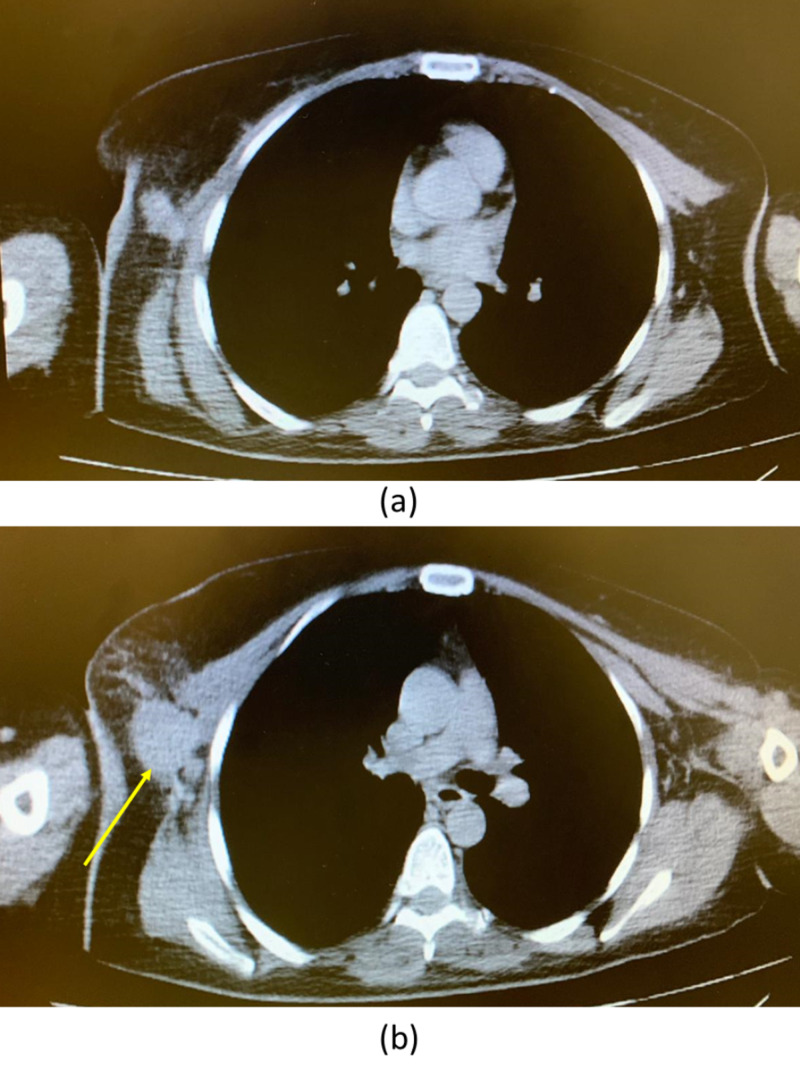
CT scan of the chest showed a 2.8-cm soft-tissue mass (yellow arrow) at the axillary right breast tail. (a) Axial cut above the lesion, and (b) axial cut through the lesion (yellow arrow).
